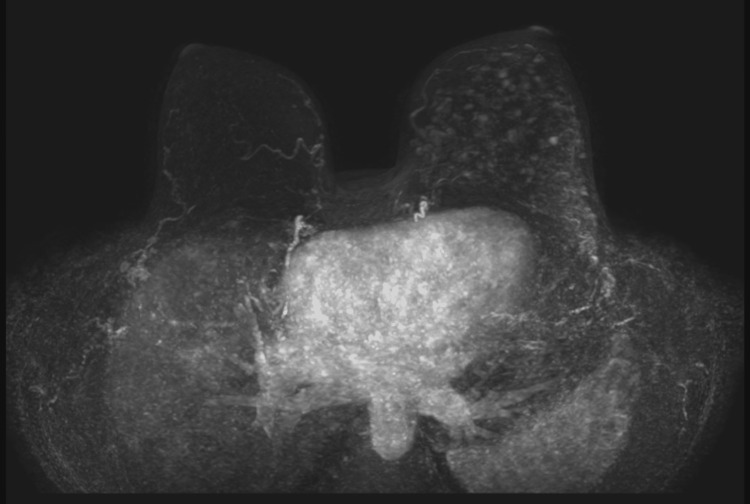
Problem-Solving Breast MRI Dynamic contrast-enhanced MRI (subtraction MIP) shows diffuse asymmetric non-mass enhancement correlating with mammographic abnormalities (BI-RADS 4).
